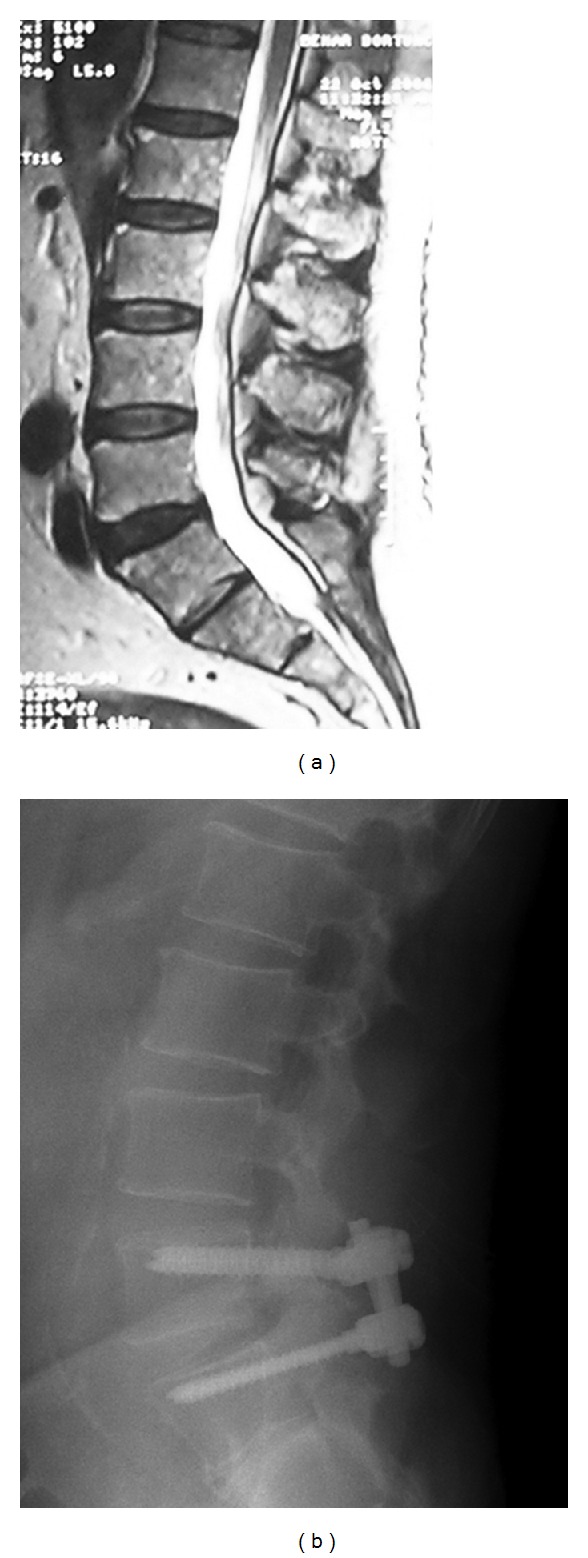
(a) A 50-year-old male complained of severe back pain attacks. (a) T2-weighted MRI scans showed degeneration of the disc at L4-L5 lumbar disc (L5- S1 considered as sacralization). (b) Following posterior transpedicular dynamic stabilization with the Cosmic system.
